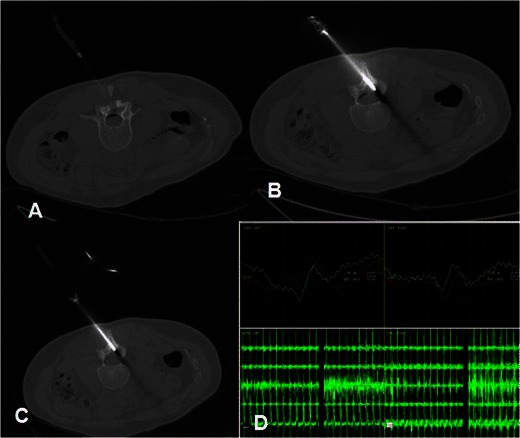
Osteoblastoma in the L2-L3 facet joint: a CT axial scan: A 22G spinal needle is inserted inside the epidural space and air is injected through the antimicrobial filter. b Axial CT scan: A trocar is drilled till the lesion. c Axial CT scan: Coaxially, a bipolar radiofrequency electrode is introduced inside the lesion and the ablation protocol is performed. d During the ablation protocol, evoked potentials are used for nerve monitoring
